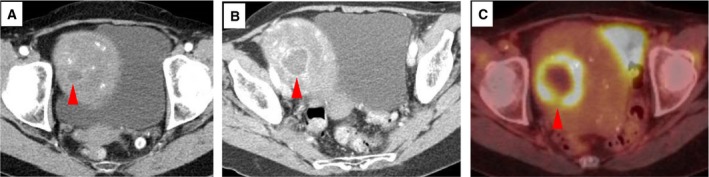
EDA detected in circulating tumor DNA (ctDNA) predicts radiographic melanoma progression. (A) Representative image from a contrast‐enhanced CT scan obtained at study entry of a 68‐year‐old female receiving pembrolizumab (anti‐PD‐1) for metastatic melanoma. Although ctDNA was detectable at this time point (and remained so thereafter), this examination was described as demonstrating no evidence of disease. However, a subtle, rim‐enhancing, centrally necrotic 1.5 cm mass (red arrowhead) is present within a large uterine fibroid. (B) Contrast‐enhanced CT image at 3 months demonstrating an interval increase in the intrafibroid lesion, now 3 cm in diameter. The CT images were again described as demonstrating no evidence of melanoma. (C) FDG‐PET/CT image at 5 months demonstrating a 5‐cm necrotic hypermetabolic lesion within a fibroid uterus. Subsequent biopsy of this mass proved the presence of metastatic melanoma.
